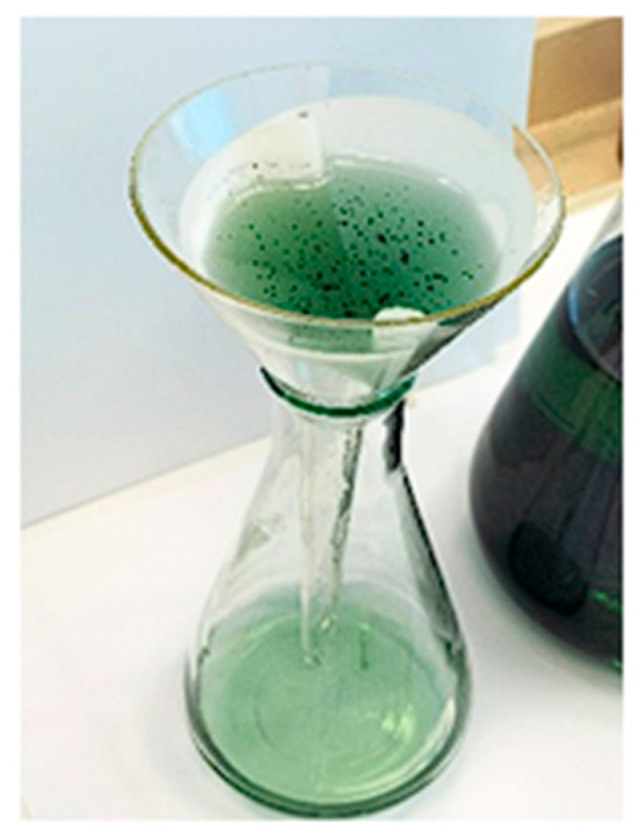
The resulting solution after ultrasonication in 1 M citric acid.
